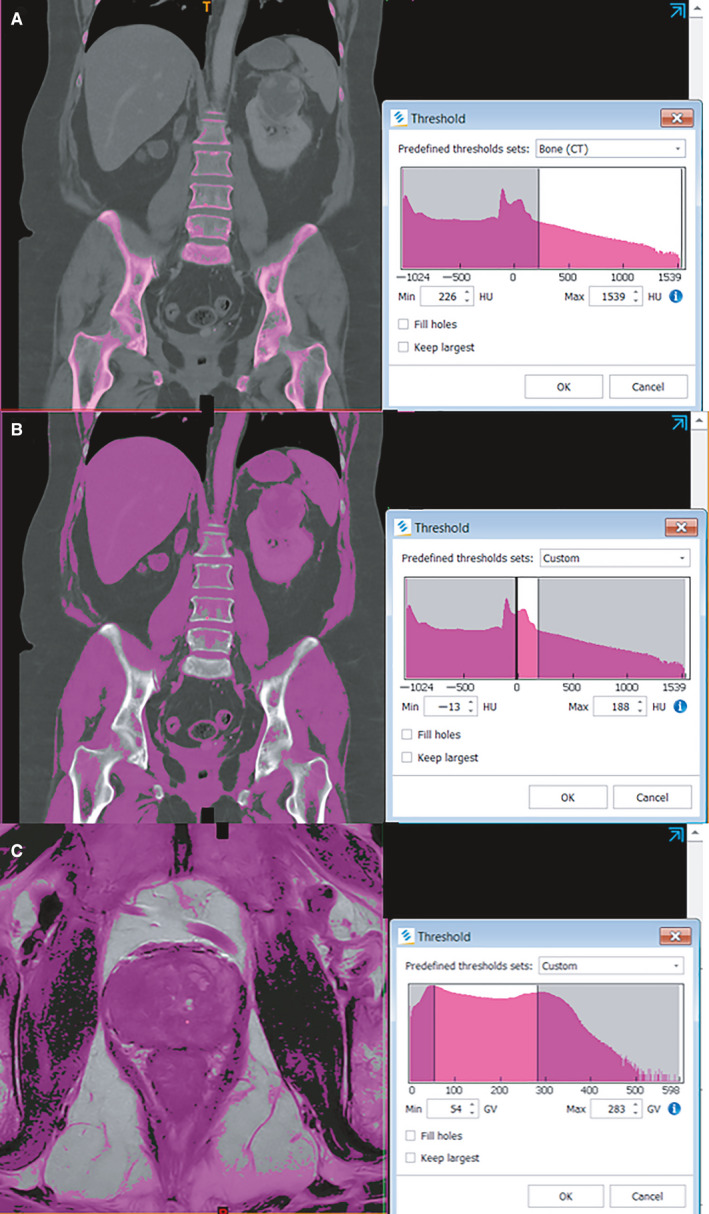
A comparison of using threshold density to initiate the segmentation process. On CT imaging of bone (A) the high density allows for rapid segmentation from surrounding soft tissue. To segment the kidney on CT (B), other soft tissues such as spleen are included but the kidney is separated from surrounding adipose tissue. To segment prostate on MRI (C) the rectum and capsular tissue are included, requiring additional manual segmentation. Screenshots taken on Mimics 21.0 (Materialise, Leuven, Belgium). The threshold for bone is predefined by the software, while the others were manually selected
